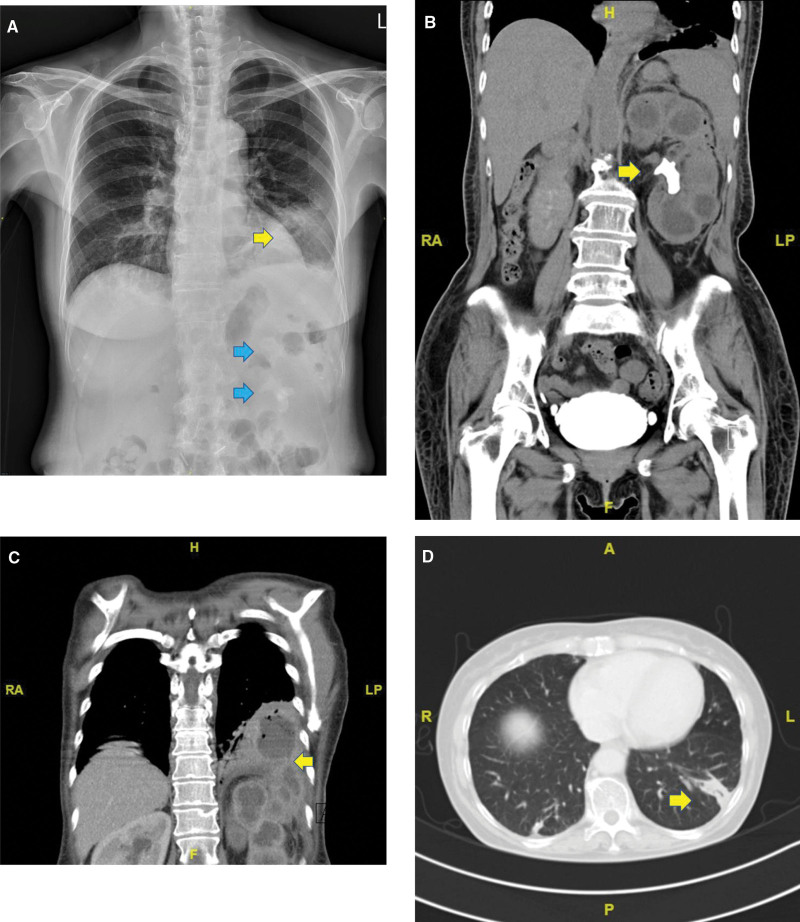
Imaging findings. (A) Chest X-ray on admission, showing left lower lung consolidation with costophrenic angle blunting (yellow arrow) and staghorn renal calculi over the left kidney (blue arrow), (B) computed tomography showing a staghorn calculus in the left kidney, with an enlarged kidney (yellow arrow), (C) computed tomography showing an abscess in the left lung and a tract of transdiaphragmatic extension (yellow arrow), and (D) computed tomography showing regression of the left lung abscess and subsegmental atelectasis 4 months after surgery (yellow arrow).
